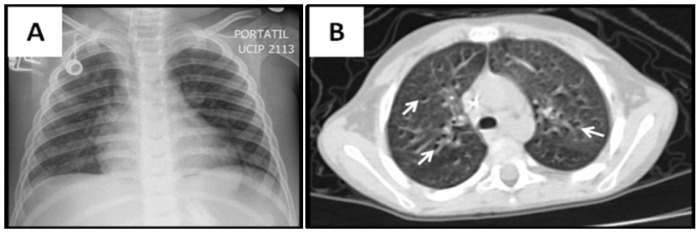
Ground-glass pattern in CMV lung infection after stem cell transplant (SCT). Four year old boy with acute lymphoid leukemia presenting with hypoxemia and bilateral rales after allogeneic SCT. Diffuse haziness is seen in CXR (A), and CT scan (B). There are interspersed airway lumen marks known as “dark bronchus” signs indicating ground-glass pattern (arrows in B).
